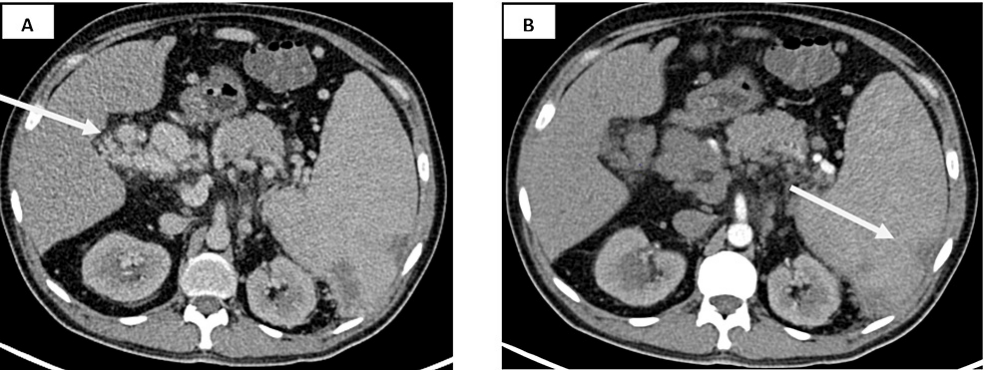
(A) Axial contrast-enhanced CT image showing numerous vascular structures adjacent to the portal vein, which enhance during the portal venous phase, suggestive of portal cavernoma. (B) Few nonenhancing hypodense-infarcted areas in the spleen.
